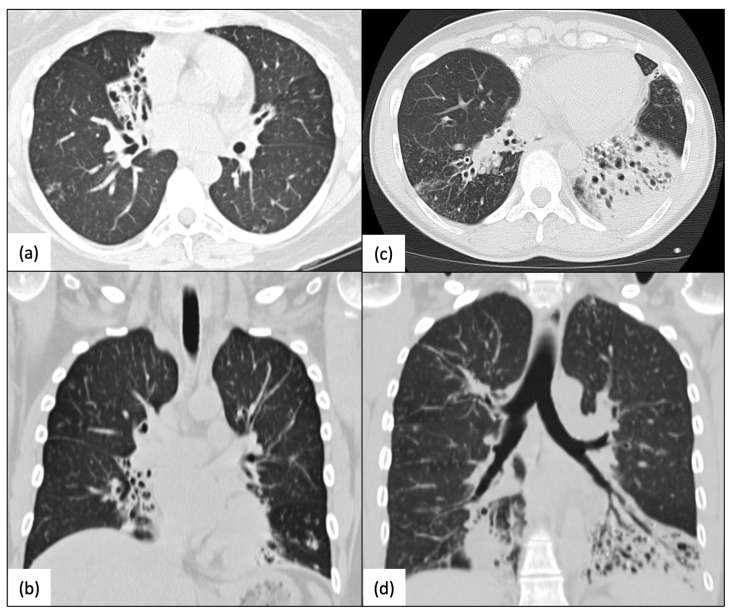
High-resolution CT scans (HRCT) of the chest. Homozygous patients with PCD for the *RSPH4A* (c.921+3_921+6delAAGT (intronic)) founder mutation. (a,b) A 40-year-old female with mild-to-moderate RML and LLL varicose bronchiectasis with a bilateral and peripheral tree-in-bud pattern, and (c,d) a 59-year-old female with increased bronchovascular markings, severe bibasilar bronchiectasis, mucus plugs, and atelectasis, more prominent at LLL. Panel organized using BioRender.com (accessed on 24 November 2022).
